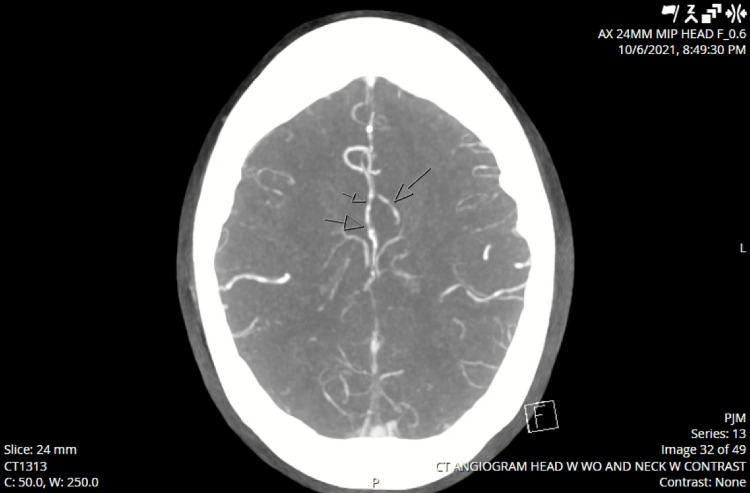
Diffusion-weighted MRI with bilateral anterior cerebral artery (ACA) distribution diffusion restriction
